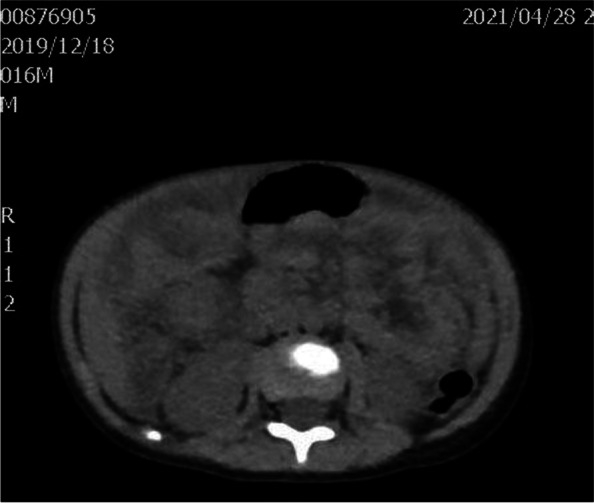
Abdominal CT scan showed localized intestinal wall thickening in the right upper abdominal cavity, thickening of the right upper abdominal omentum, extensive lymphadenopathy and ascites
